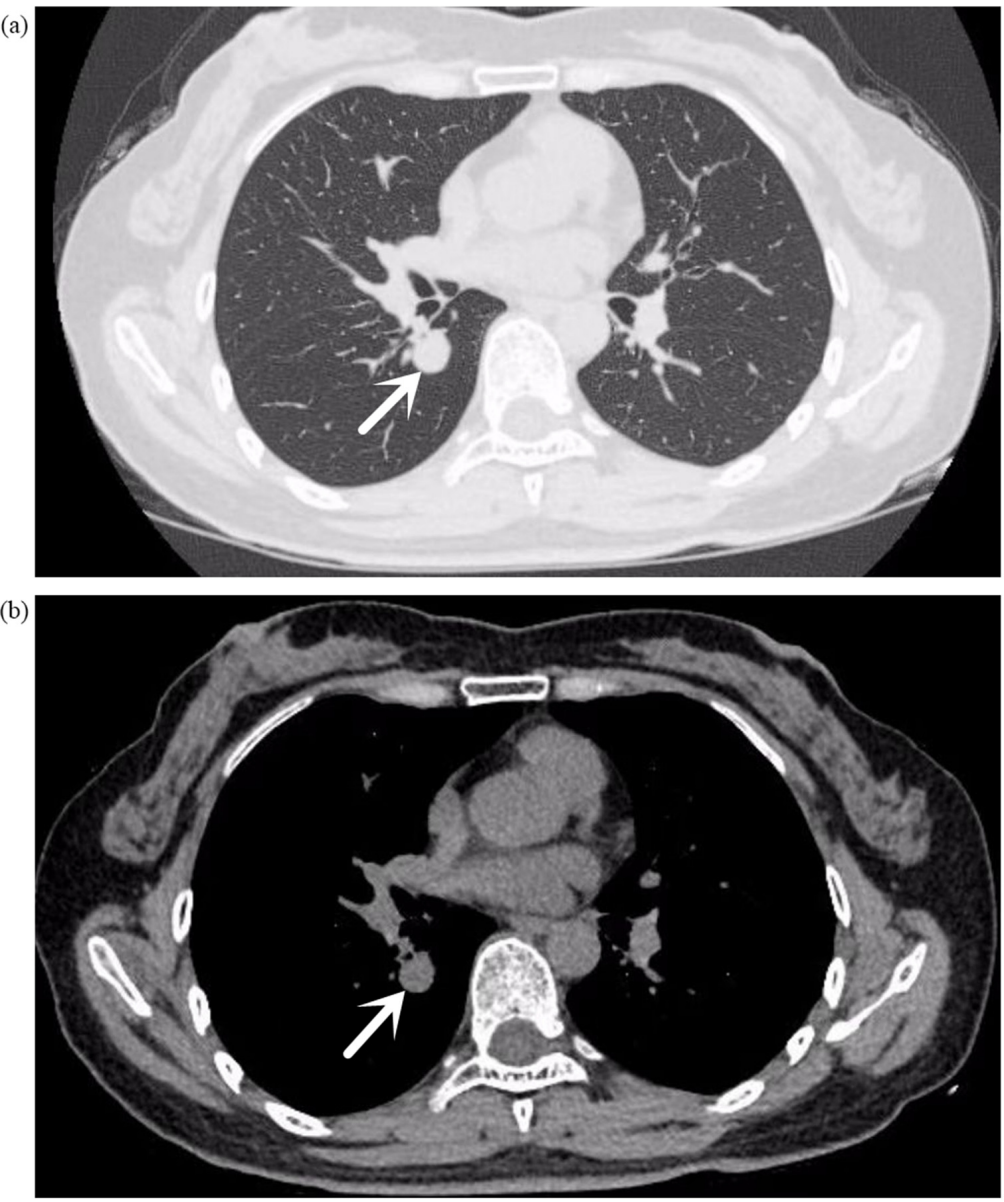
(a) (Lung window), (b) (mediastinal window) chest plain CT: small nodules in the dorsal segment of the right lower lobe, with smooth margins. The arrow indicates the location of the tumor.
